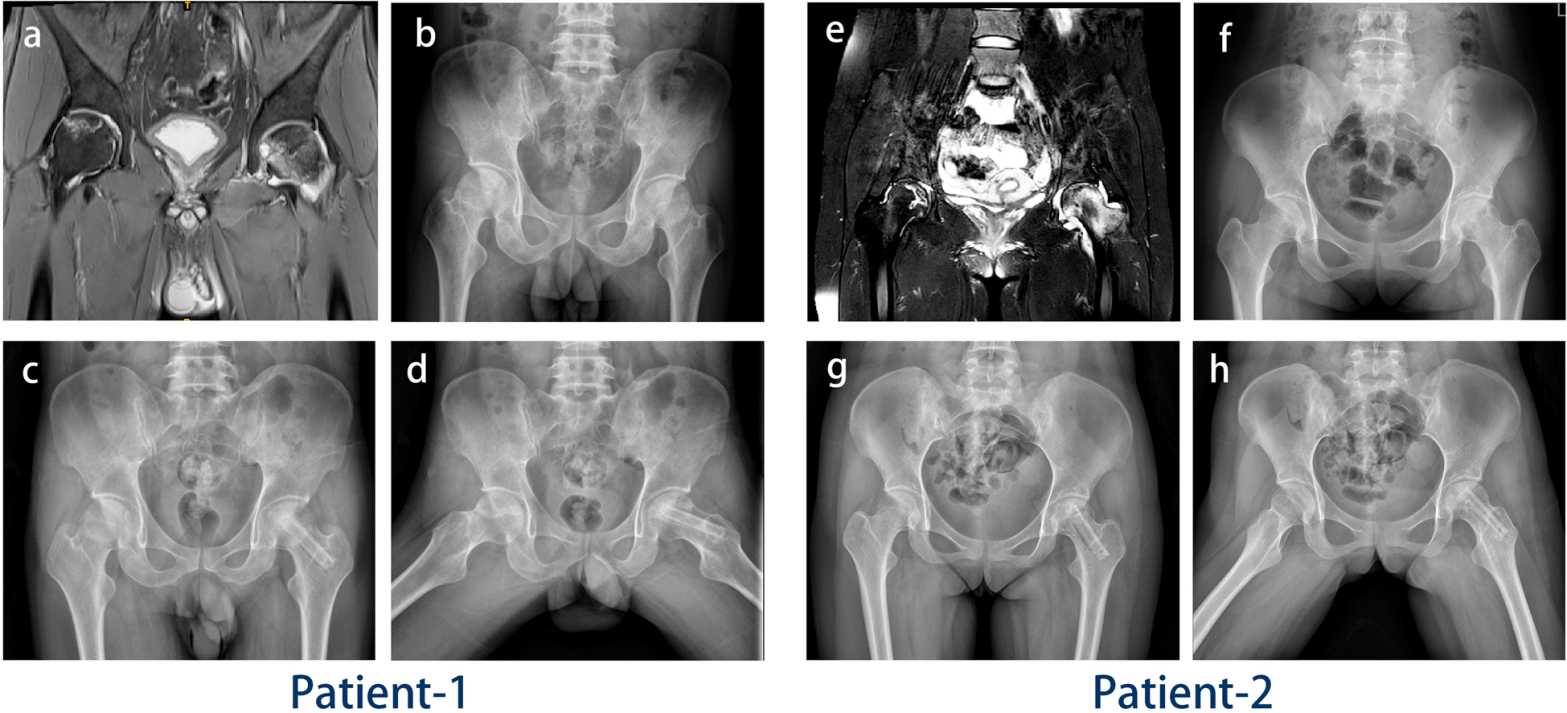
Two patients’ pre- and postoperative images. a–d Patient 1,the case in the left hip was classified as JIC C1 NONFH. e–h Patient 2, the case in the left hip was classified as JIC C2 NONFH. The top left panels (a, e) show the preoperative T2-weighted MRI scan. The top right panels (b, f) show the preoperative X-ray. The bottom panels show the postoperative X-ray, including patient 1’s left hip after the LBT at the 4-week follow-up (c, d) and patient 2’s left hip after the LBT at the 4-week follow-up (g, h).
